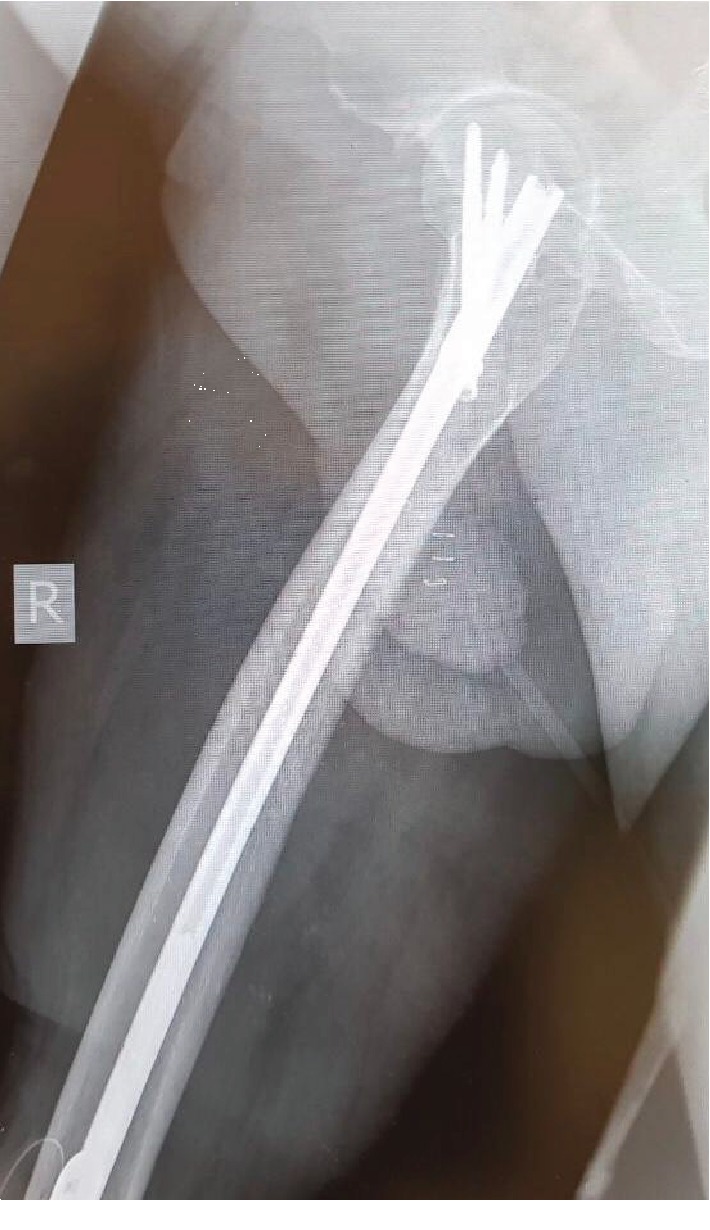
Radiograph shows femur with proximal locking in lateral view.
